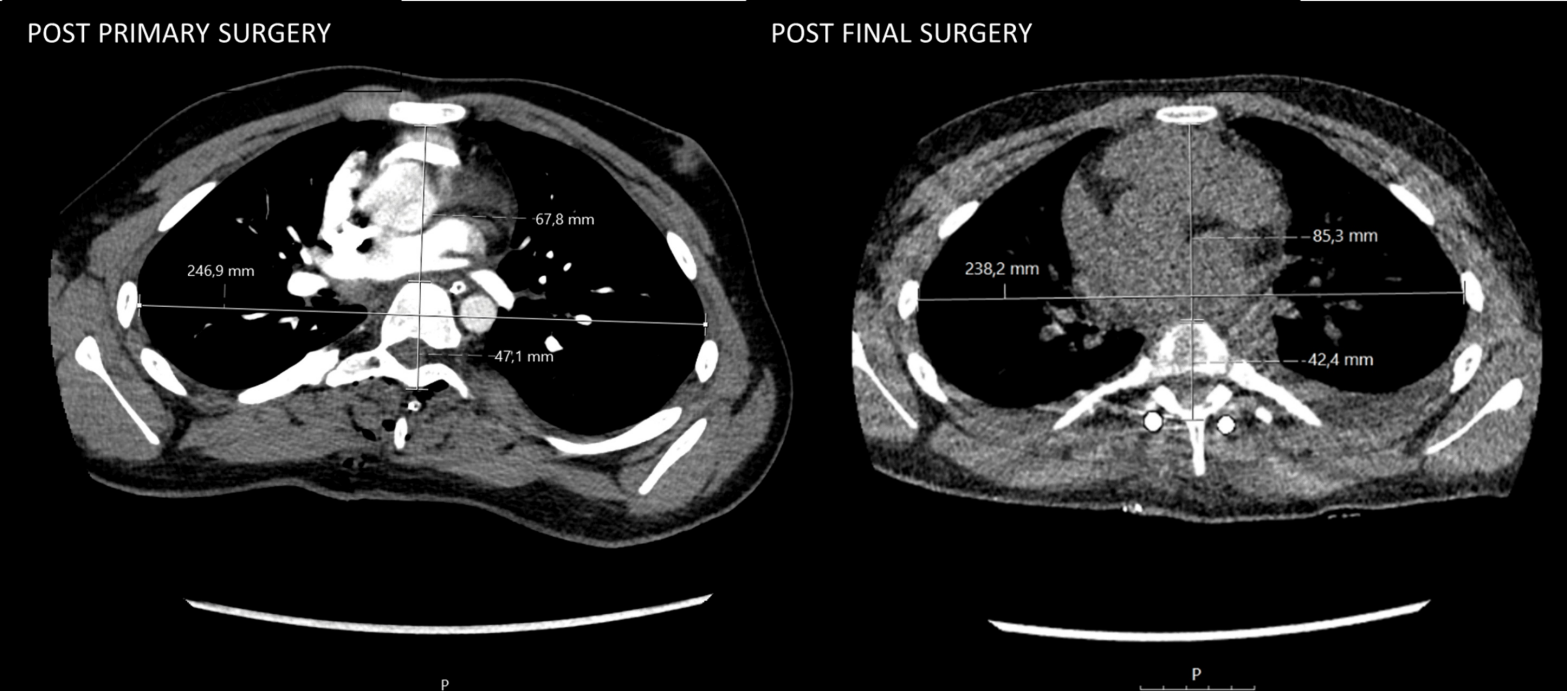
After initial surgery (left) and post final stage surgery (right) chest CT examinations. Measures for calculation of Spinal Penetration Index Surface (SPIS) and Modified Haller Index (MHI).
